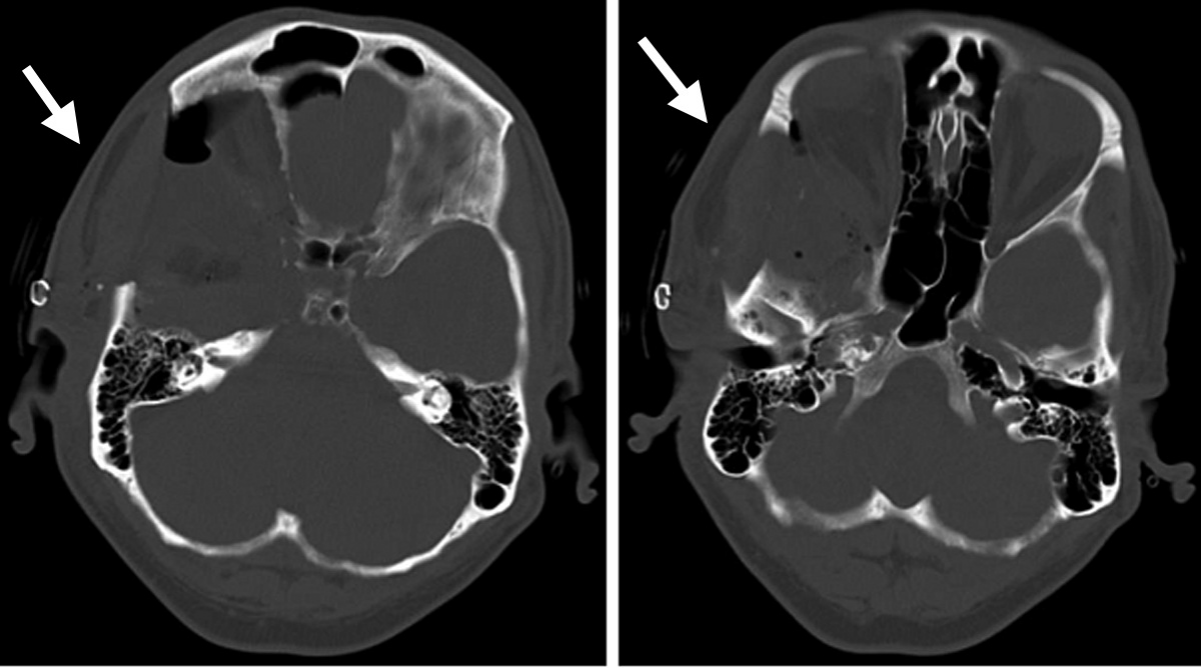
Postoperative computed tomography scan bone window images Axial cuts showing complete removal of the sphenoid bony hyperostosis, lateral and superior orbital wall, clinoid, and floor of the middle cranial fossa (arrows).
